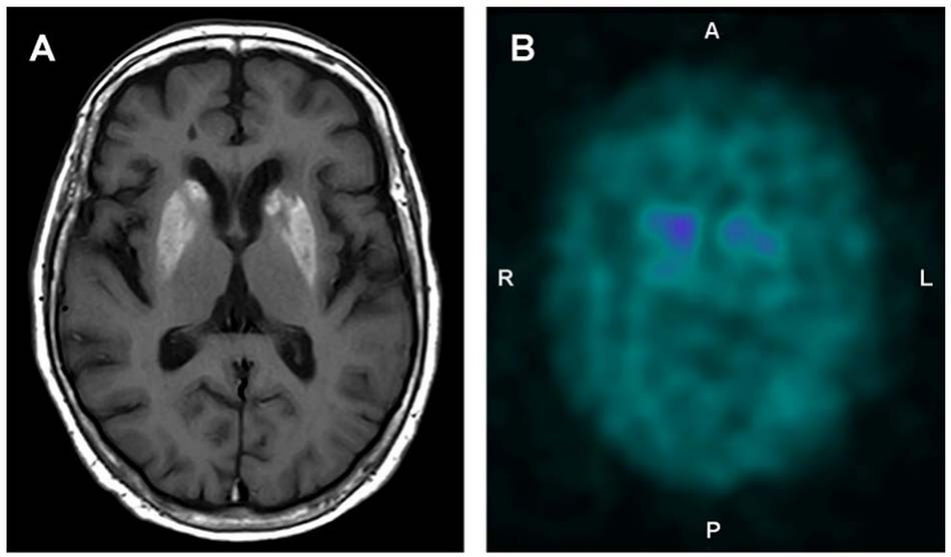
Magnetic resonance images of the brain of a 78-year-old woman with hyperglycemic chorea revealing (A) hyperintensity on T1-weighted image in the basal ganglia bilaterally. (B) Dopamine transporter single-photon emission computed tomography showing marked reduction of striatal accumulation bilaterally.
